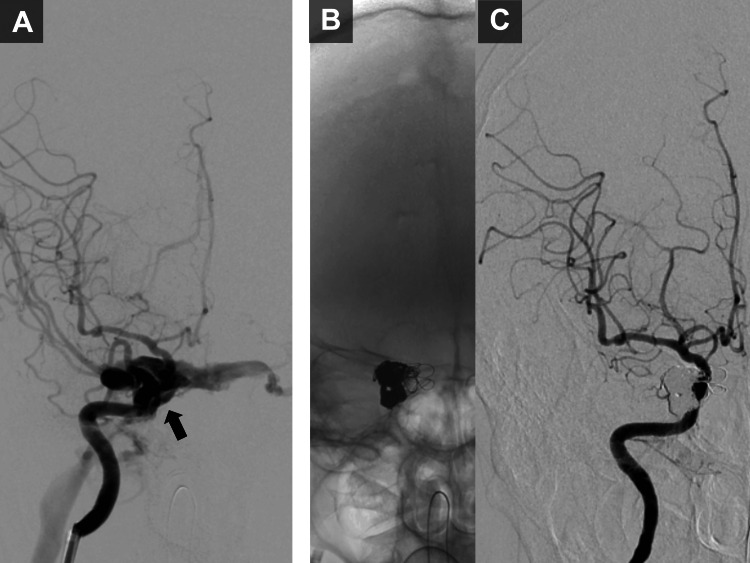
Endovascular treatment of a direct carotid-cavernous fistula. (A) Anteroposterior right internal carotid artery (ICA) angiography demonstrating a high-flow direct carotid-cavernous fistula (CCF) (black arrow). (B) Anteroposterior radiograph after embolization showing coils within the right cavernous sinus. (C) Anteroposterior right ICA angiography after embolization demonstrating complete occlusion of the CCF.
